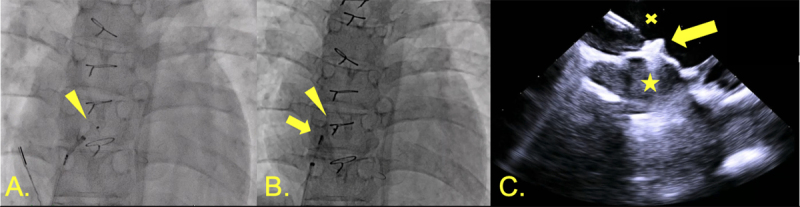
Fontan fenestration closure. (A) Left disc of 4-mm Amplatzer ventricular septal defect closure device deployed in common atrium (triangle). (B) Right disc now also deployed in Fontan (arrow). (C) Intracardiac echocardiography showing device deployment (arrow) across fenestration from common atrium (star) to Fontan (x).
